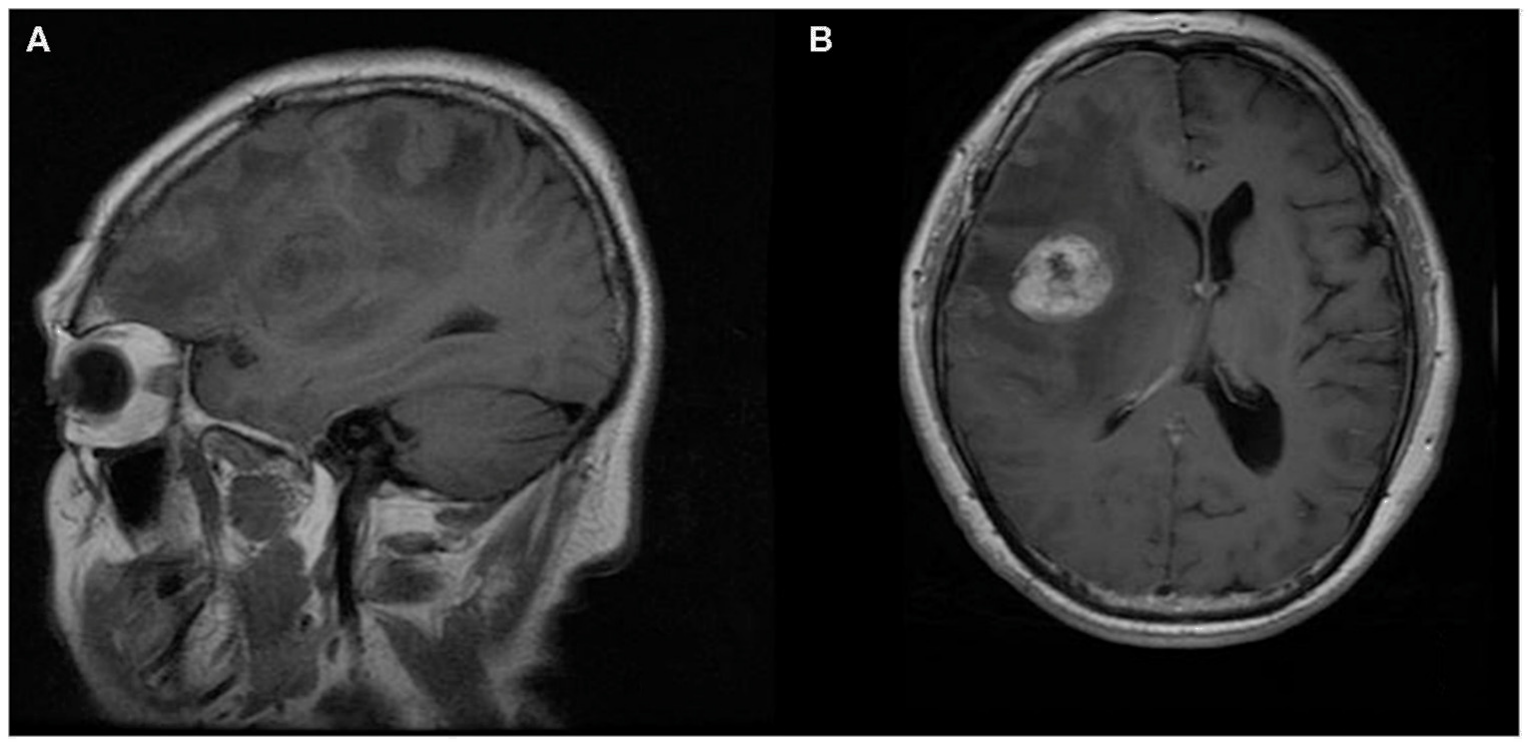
A 70-year-old man with supratentorial intraparenchymal anaplastic ependymoma in the right frontal lobe on (A) T1-weighted image and (B) contrast T1-weighted image. The tumor appears as heterogeneous signal intensity and marked peritumoral edema.
